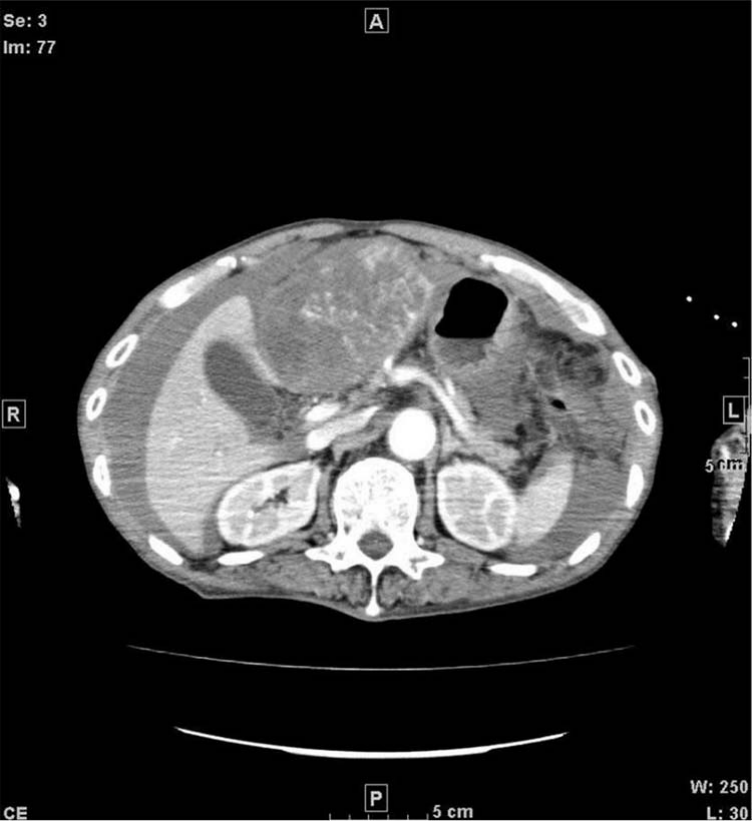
CT when the patient was transported in a state of shock shows increased bloody ascites but no apparent extravasation. CT, computed tomography.
